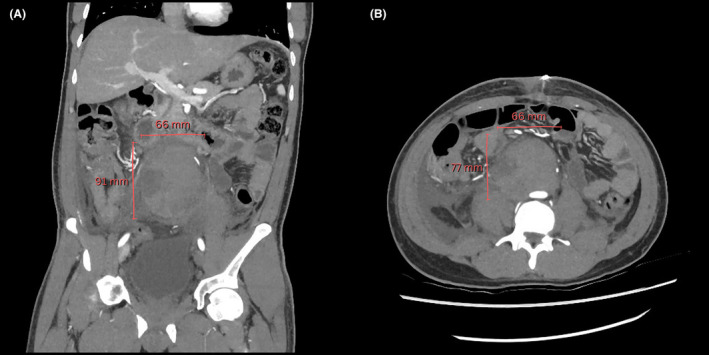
Coronal (A) and axial (B) abdominal CT angiography images showing the large non‐enhancing retroperitoneal hematoma (91 × 66 × 77 mm), extending from the common iliac bifurcations to the level of the inferior mesenteric artery at L3. The collection shows no contrast enhancement.
